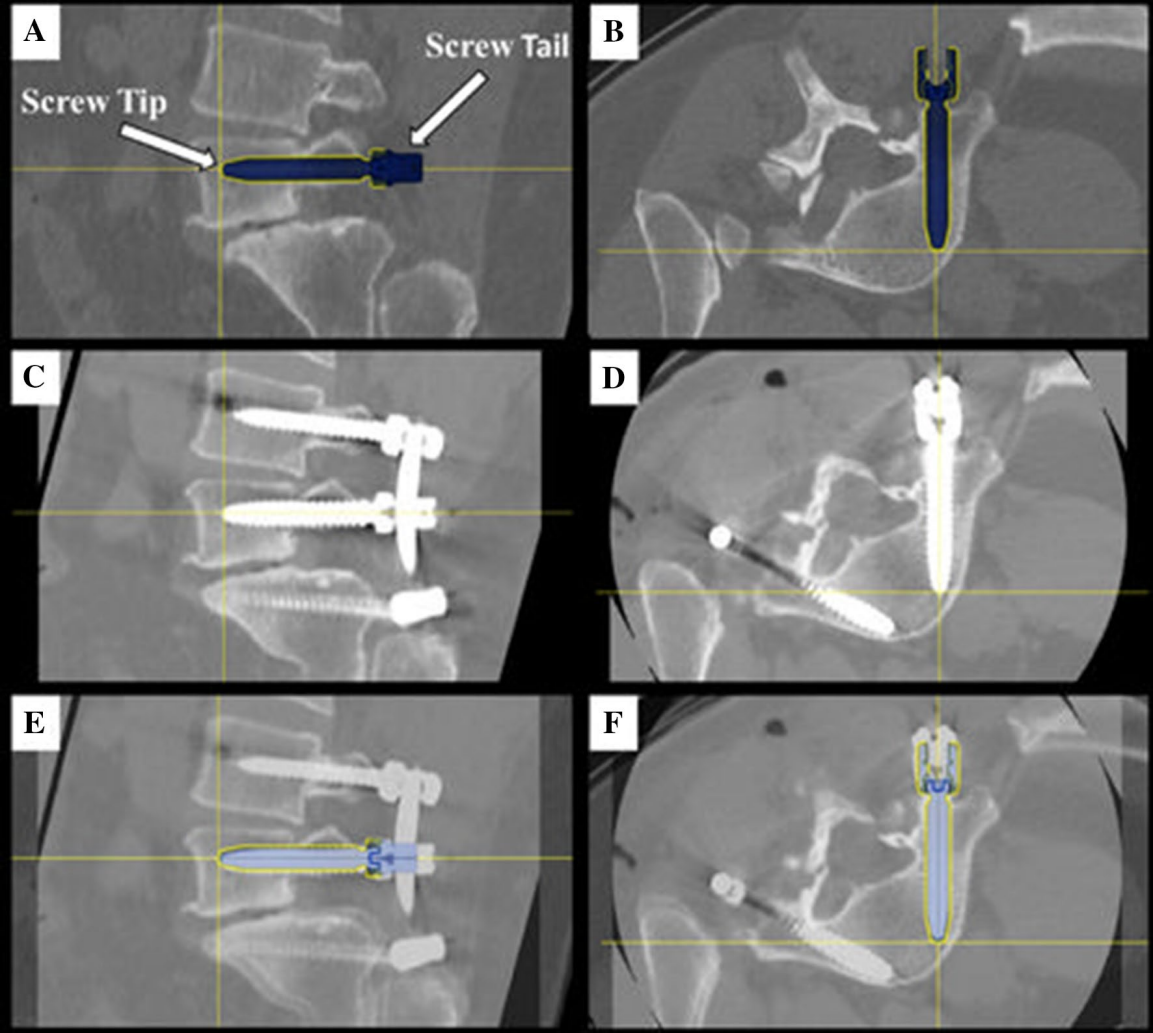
Screw tip, tail, and angle offset assessment. Right L5 screw planning in (a) sagittal and (b) axial planes. Image overlay analysis with preoperative planned trajectory and postoperative screw placement in (c) sagittal and (d) axial planes. Postoperative CT of L5 screw placement without a medial or lateral breach in (e) sagittal and (f) axial planes. The crosshairs indicate screw tip
